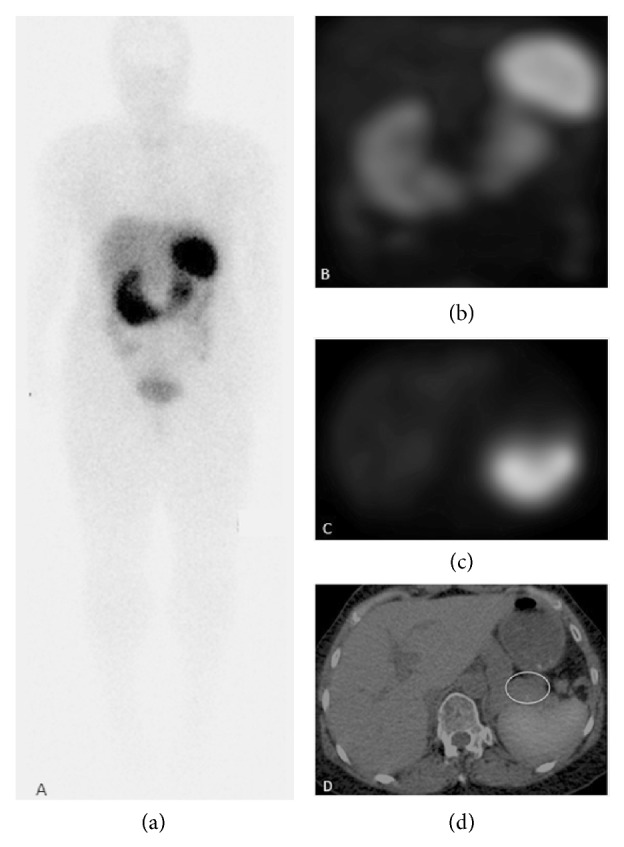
24-hour ^111^In-pentetreotide scan. Anterior whole body planar image (a), MIP reconstruction of SPECT acquisition (b), axial SPECT image (c), and fused axial SPECT/CT image (d) show normal biodistribution of the radiotracer and no uptake in the pancreatic tail mass, ruling out a splenule.
